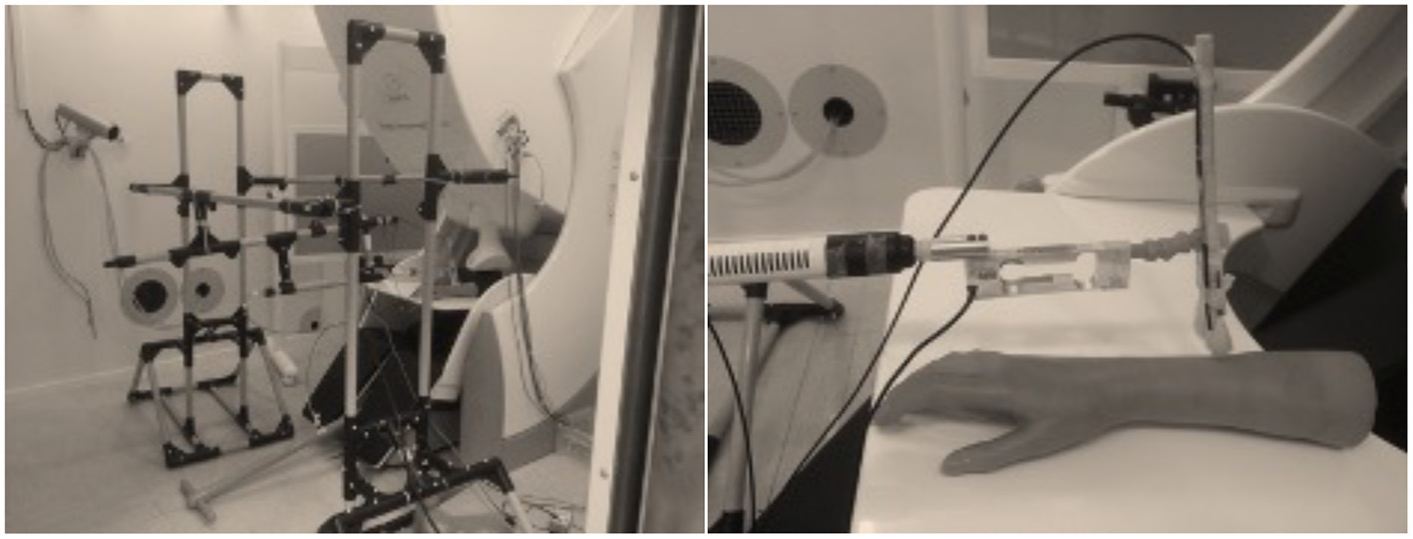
Left: The brush robot at NatMEG (Karolinska Institutet, Stockholm, Sweden) used to study gentle touch, that is, slowly conducting tactile (CT) fibers (57). Right: The brush robot uses similar PAMs as the PAM stimulator as the device in the Figure 4. Note that the computer-controlled device contains two PAMs for lifting and moving the brush, load cell to measure the force applied on the skin, accelerometer to monitor the movement, and two multifilament optic fibers to pick up the velocity of the brush movement and skin contact of the brush.
